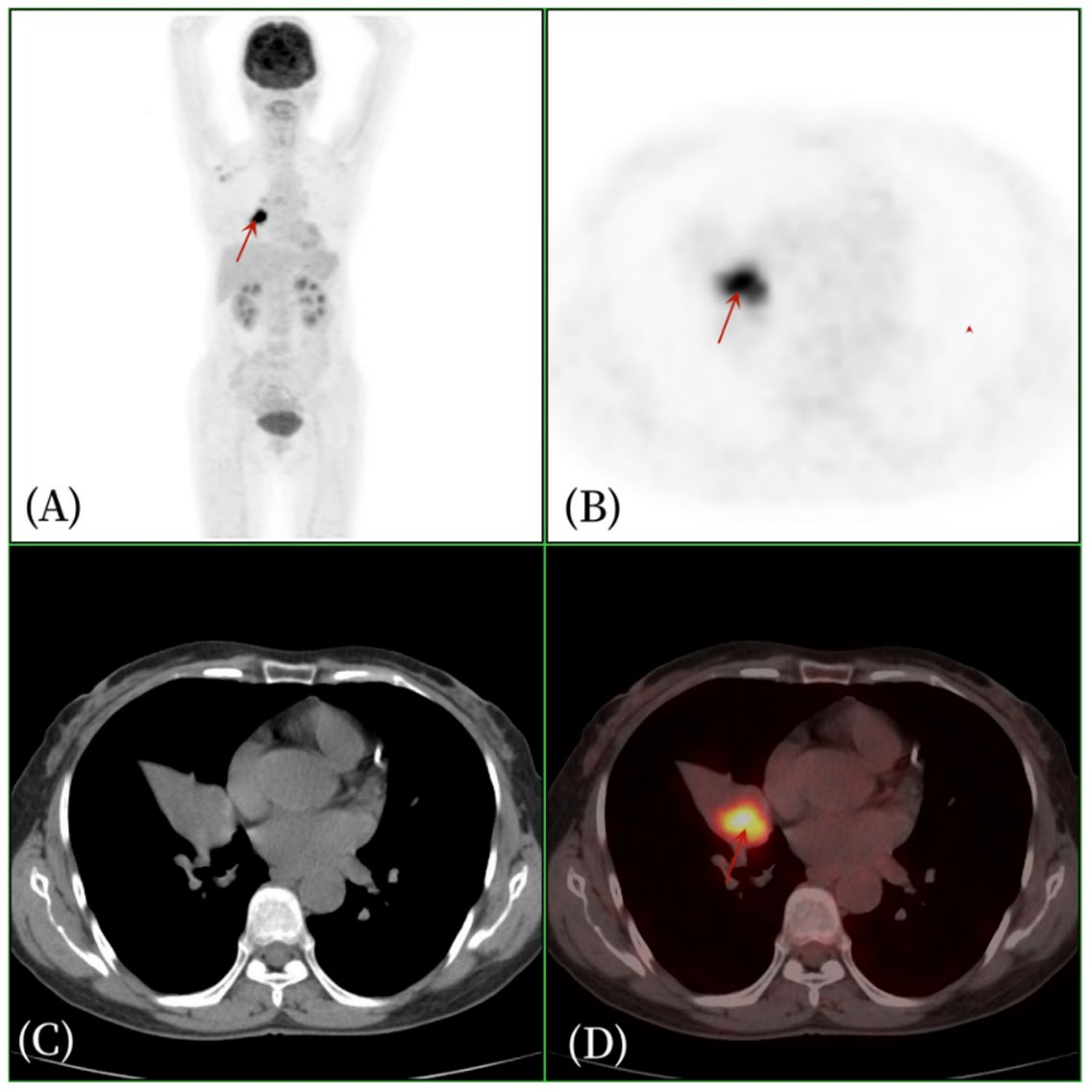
Female, 57 years old, pulmonary angiomatoid fibrous histiocytoma. (A) whole body MIP, (B) axial PET, (C) axial CT, (D) axial fusion. The 18F-FDG PET/CT revealed right middle lobe atelectasis and a 2.2 cm × 2.5 cm soft tissue density nodule within the bronchus of the right middle lobe of the lung. The density was uneven, with clear boundaries, and there was increased glucose metabolism, with an SUVmax of 11.2 (arrow).
